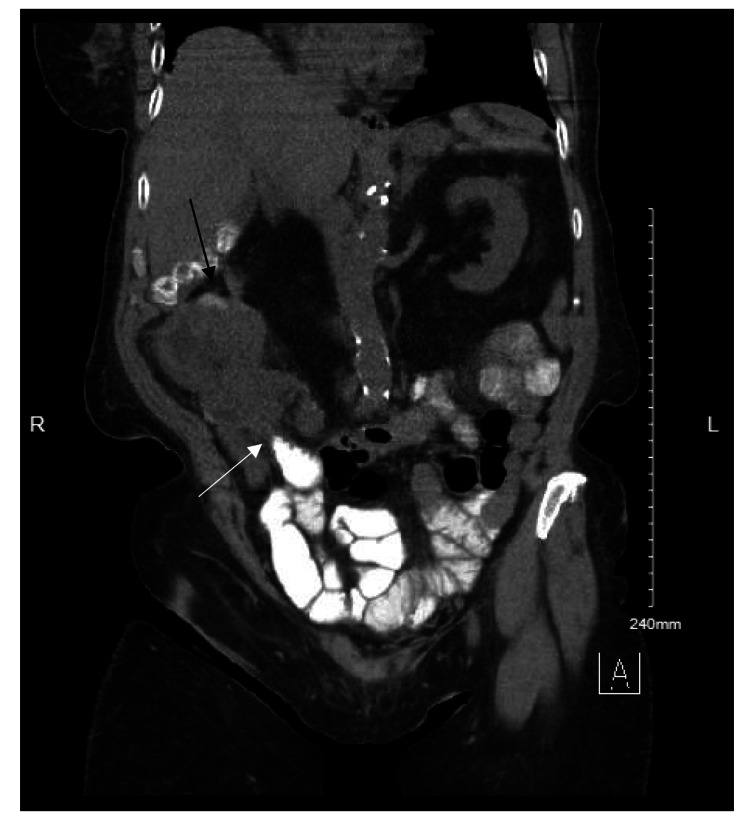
Coronal CT scan of the abdomen demonstrating ileocecal intussusception (black arrow). Notably, oral contrast appears to be stagnant at the site of intussusception (white arrow), unable to pass beyond the obstructed segment, further confirming the mechanical obstruction.
